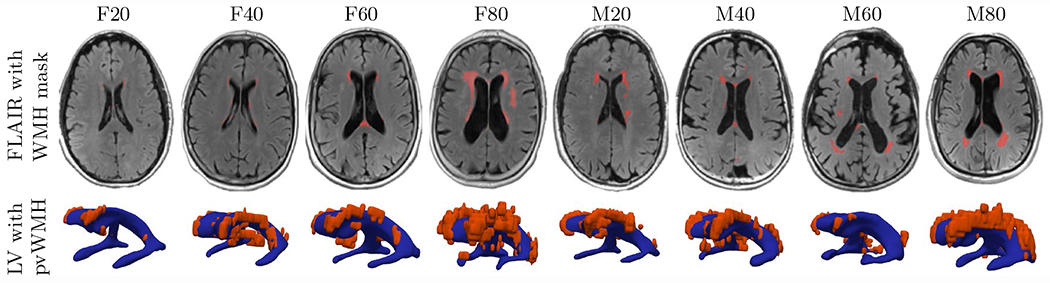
Representative axial FLAIR images for each of the eight different subjects with a white matter hyperintensity (WMH) mask (in red) in the first row, and the corresponding reconstruction of the lateral ventricles (LV) (in blue) and the pvWMHs (in red) in the second row. F20 through F80 and M20 through M80 stand from female/male subjects from the 20th through the 80th percentile with respect to total CSF volume (color figure online)
